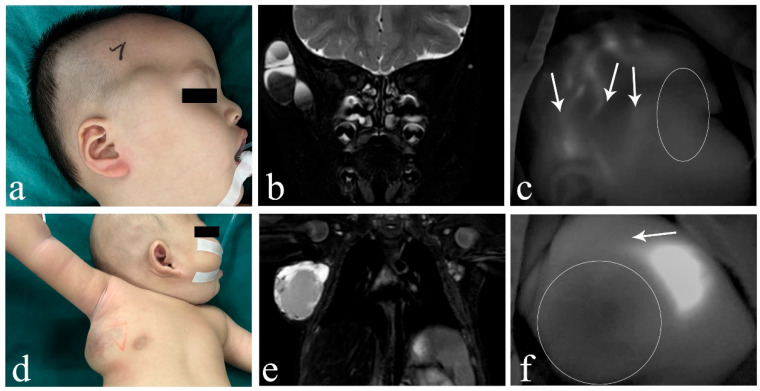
Images of macro/mixed cLM with ICH. (a) A 2-year-old male patient with macro cLM of the right temporal region. (b) Preoperative MRI indicated macro cysts with ICH. (c) Intraoperative ICG lymphography finding without any inflow draining into the lesion. (d) A 2-month-old male patient with mixed cLM of the right chest. (e) Preoperative MRI indicated mixed cysts with ICH. (f) Intraoperative ICG lymphography finding without any inflow draining into the lesion. *Arrow head*, lymph vessel; *circle*, region of the lesion. cLM, cystic lymphatic malformation; ICH, intracystic hemorrhage; MRI, magnetic resonance imaging; ICG, indocyanine green.
